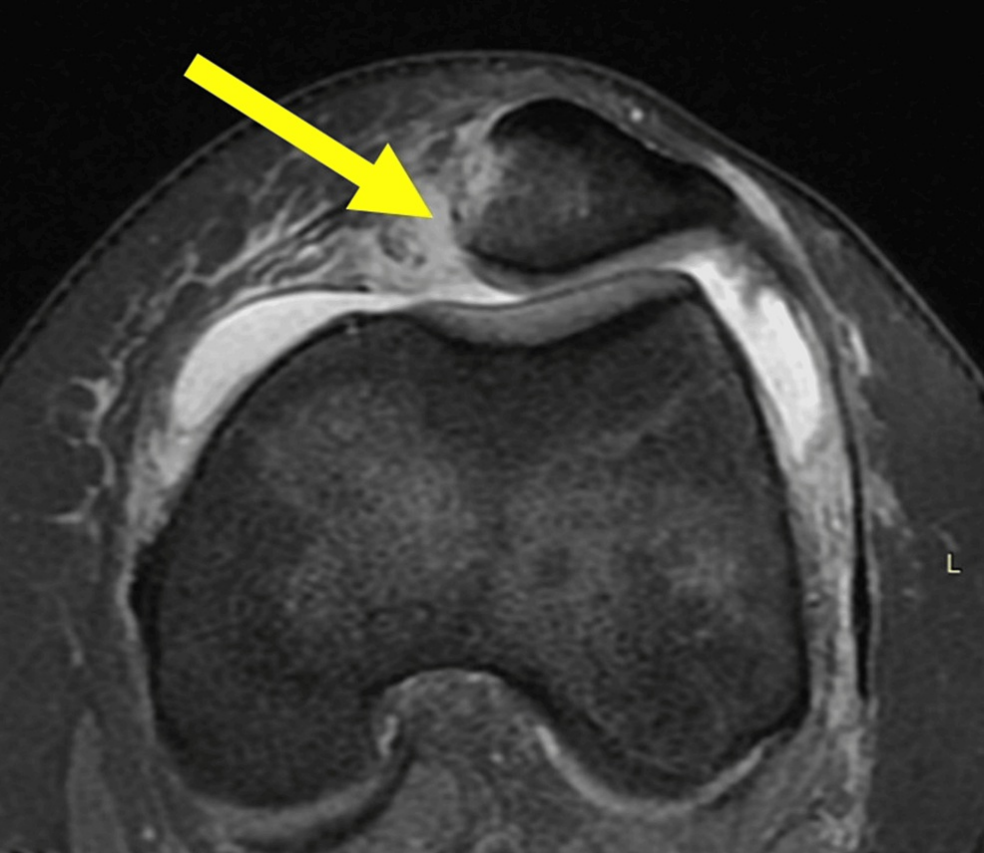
MRI of the left knee of a 13-year-old girl with acute PD Arrow indicates patellar avulsion of MPFL (arrow). Figure created by Panagiotis Samelis.
